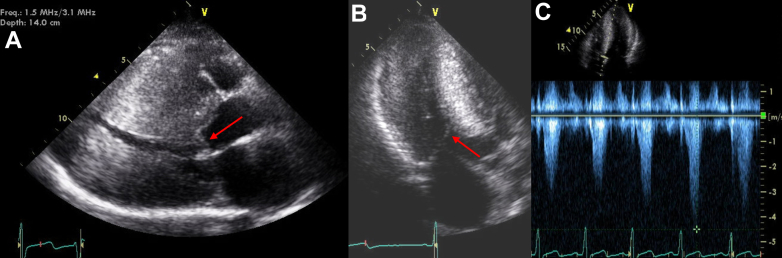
Systolic Anterior Motion of the Mitral Valve With Obstruction The patient's echocardiographic images at age 19. Parasternal long-axis (A) and apical 3-chamber (B) views in systole demonstrate septal contact of the mitral valve (red arrows). LV outflow gradient >80 mm Hg with Valsalva (C). LV = left ventricle.
